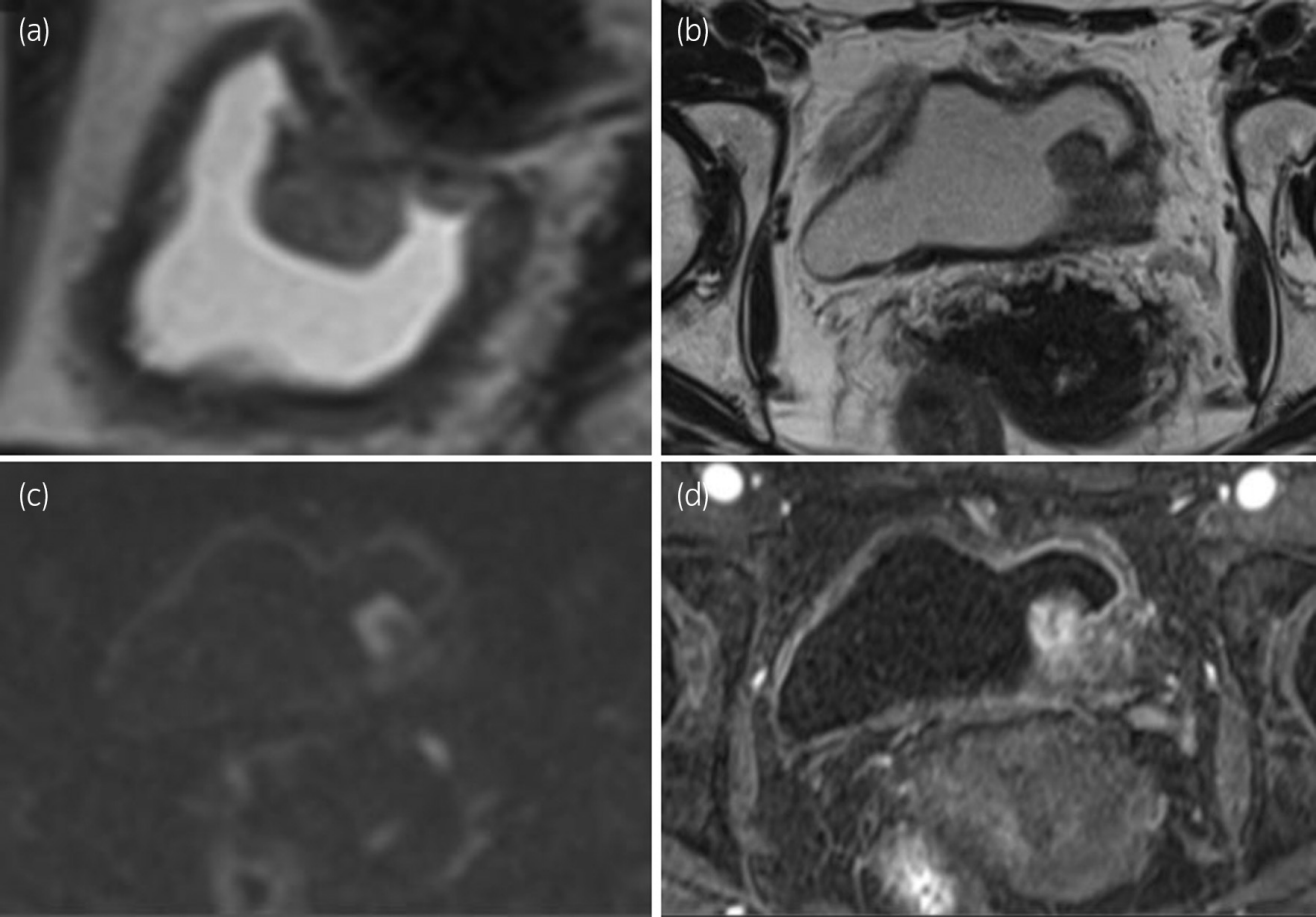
MRI images of cT3N0M0 bladder cancer before TURBT: (a) sagittal view of the T2‐WI image; (b) axial view of the T2‐WI image; (c) axial view of the DWI image; (d) axial view of the contrast‐enhanced image.
